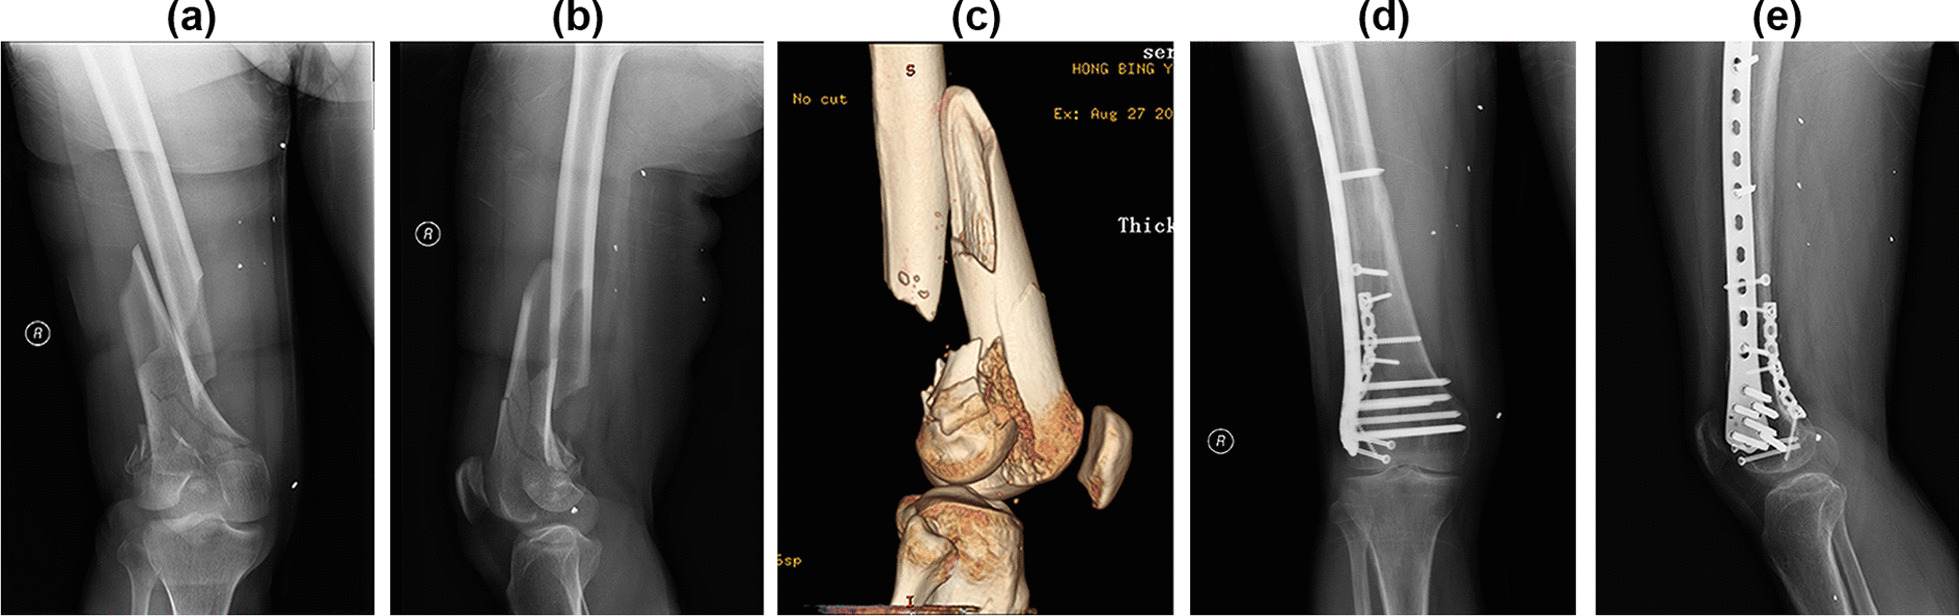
A 52-year-old man was injured on a motorcycle. a, b Preoperative anterior and lateral X-rays showed a fracture of the lower segment of the right femur and fracture of the right femoral condyle; c The three-dimensional CT showed a fracture of the lower right femur and coronal shear fracture of the right lateral femoral condyle; d, e Positive and lateral X-ray films showed fracture healing 3 months after the operation
